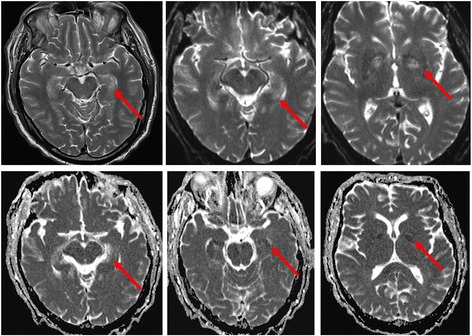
Magnetic resonance imaging sequences show focal hypoxic ischemic brain injury (HIBI) within the hippocampi and basal ganglia bilaterally. The images shown represent the acute changes after HIBI within the first week after resuscitation. In the top row, T2-weighted sequences reveal abnormal signaling in the hippocampi and basal ganglia as highlighted by the *red arrows*. In the bottom row, restricted diffusion-weighted imaging confirms HIBI in the affected regions of the hippocampi and basal ganglia as highlighted by the *red arrows*
